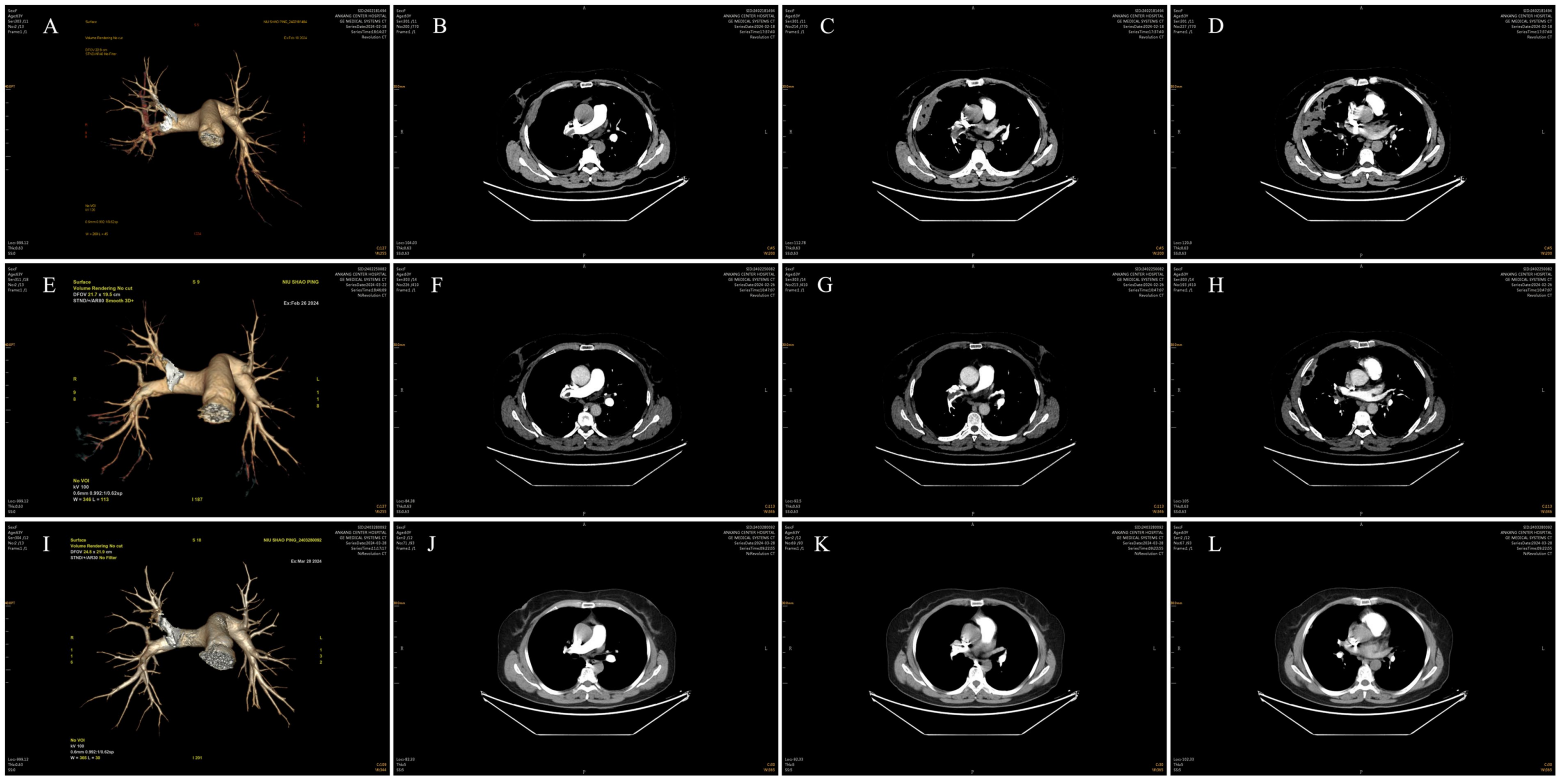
Pulmonary artery CTA. (A–D) Pulmonary artery CTA showed emboli in the main trunk of the right pulmonary artery and its branches in the upper, middle, and lower lobes of the right lung. (E–H) Pulmonary artery CTA demonstrated slight resorption of emboli in the main trunk of the right pulmonary artery and its branches in the upper, middle, and lower lobes of the right lung compared to previous images. (I–L) Pulmonary artery CTA showed a reduction in emboli in the main trunk of the right pulmonary artery and its branches in the upper, middle, and lower lobes of the right lung compared to previous images.
